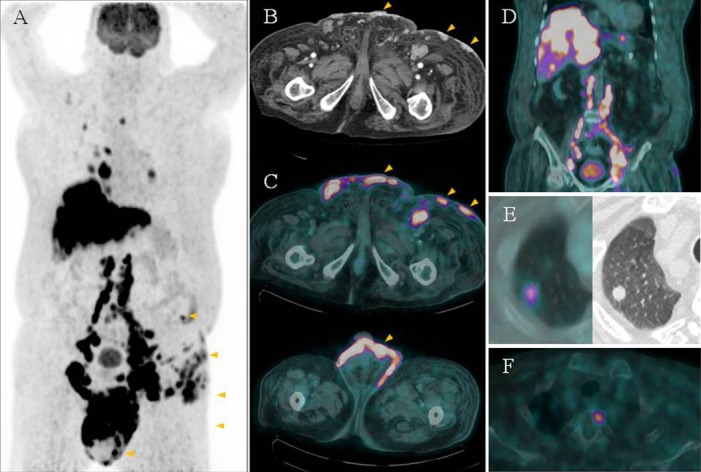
A 96-year-old man underwent F-18 FDG PET/CT to determine the initial clinical stage of his eccrine carcinoma. A maximal intensity projection image showed intense FDG uptake in multiple sites including the skin (arrowheads), nodes, liver, and lung. Axial enhanced CT (B) and fusion PET/CT (C) findings revealed varying degrees of FDG uptake over the groin, scrotum, penis, left pelvic wall, left hip, and left thigh (SUV_max_ 3.1-13.9), corresponding to enhancing skin thickening and nodules visualized on CT. D-F multiple FDG-avid distant metastatic lesions were also evident in the liver, lung, and bone.
